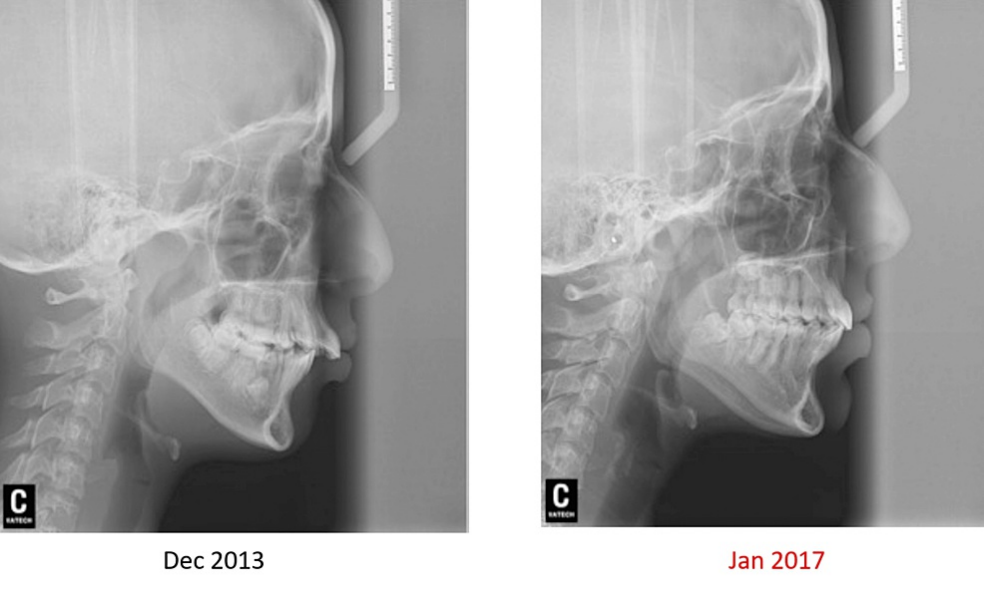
Initial and final lateral cephalometric radiographs
